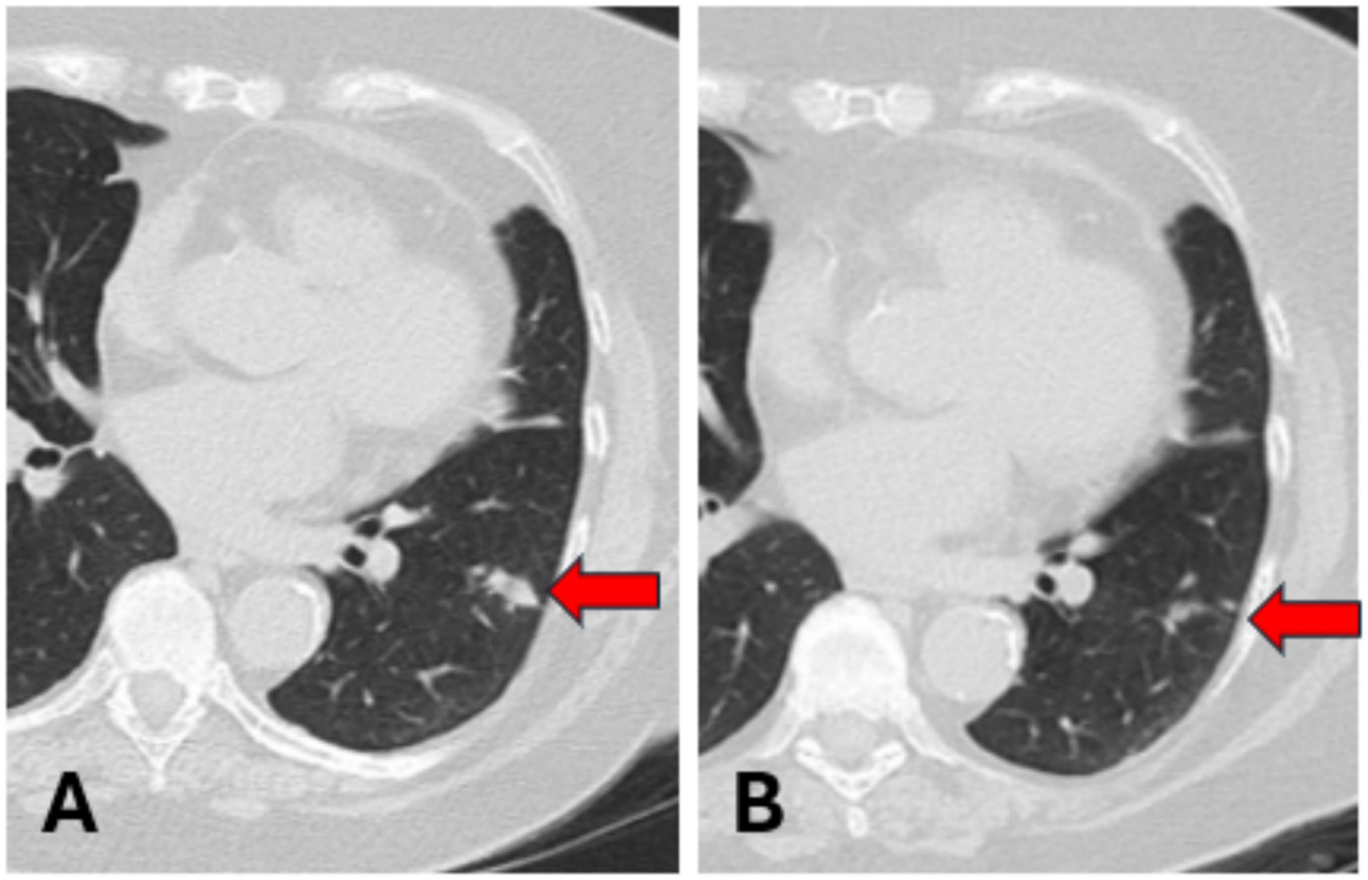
(A) Pre-treatment CT scan. (B) CT scan after 3 months of azithromycin therapy.
